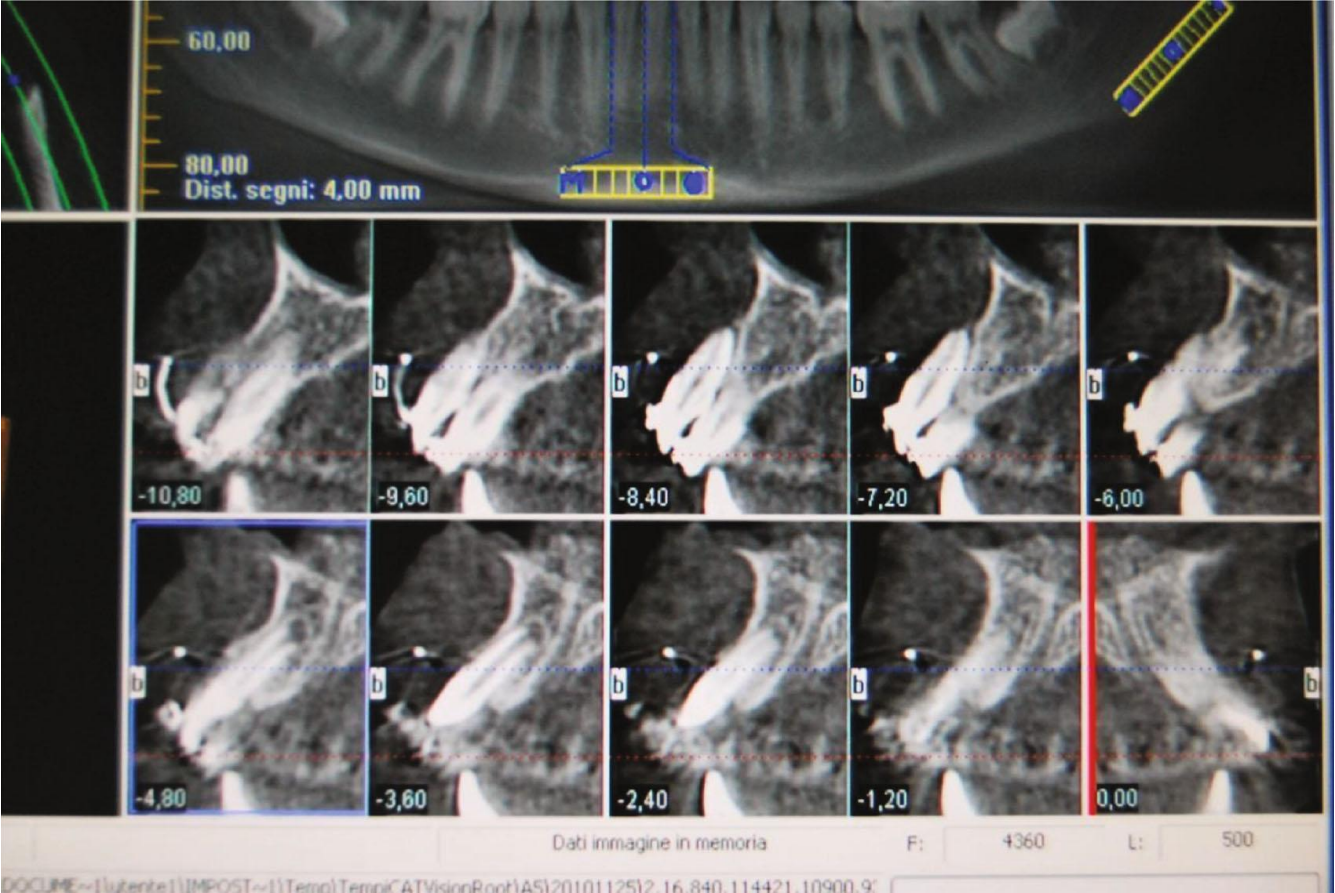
TC cone beam shows the lack of vestibular bone tissue around the permanent 1.1.
